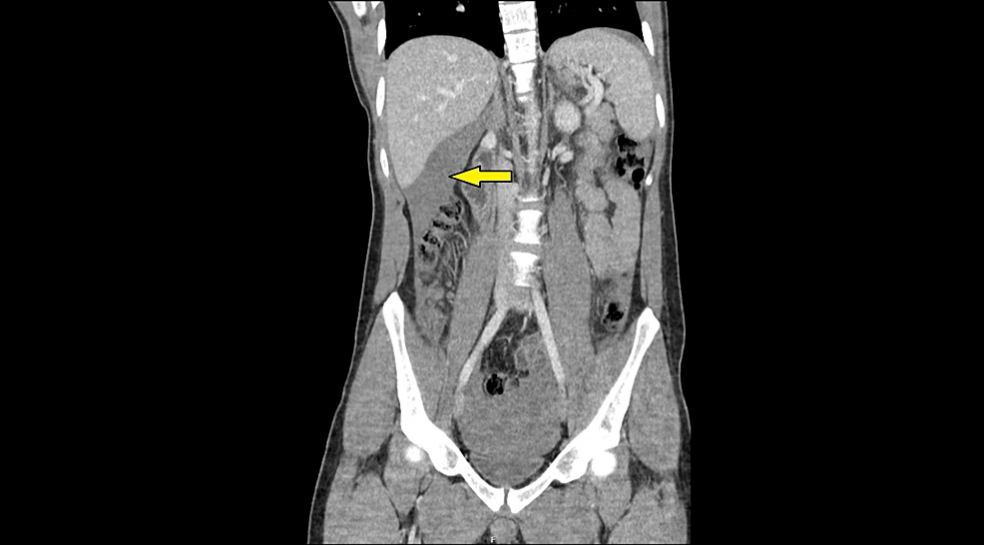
A coronal CT image of the abdomen and pelvis demonstrating high-density free fluid in the right paracolic gutter (denoted by a yellow arrow)
